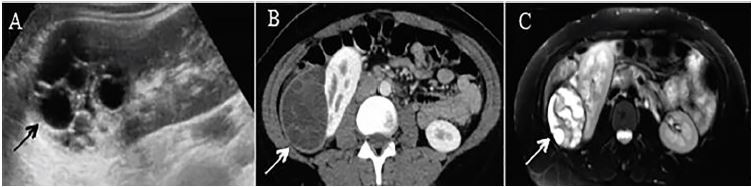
Large, subscapular multicystic renal mass with internal septations as indicated by the arrow on (A) renal ultrasound, (B) computerized tomography, and (C) magnetic resonance imaging T2.
